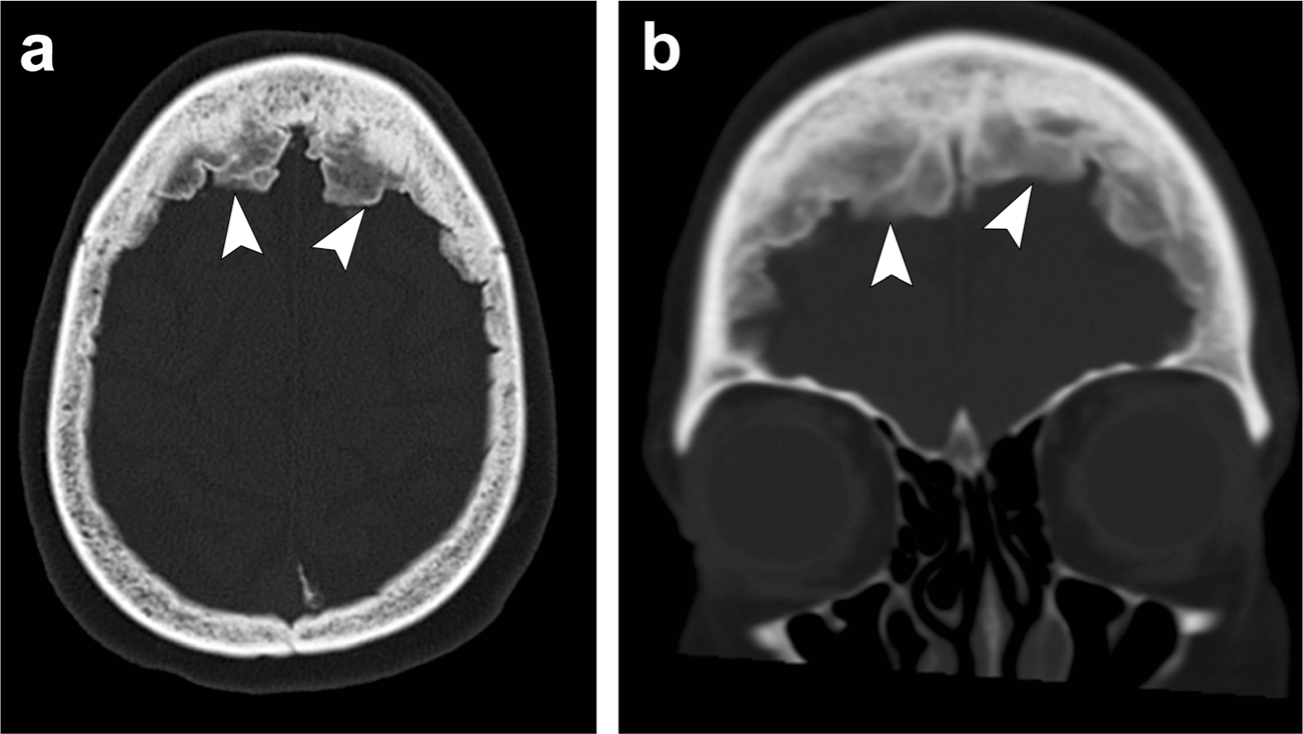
Hyperostosis frontalis. Axial (a) and coronal (b) head CT images portray irregular thickening of the inner table of the frontal bone consistent with hyperostosis frontalis (*arrowheads*)
